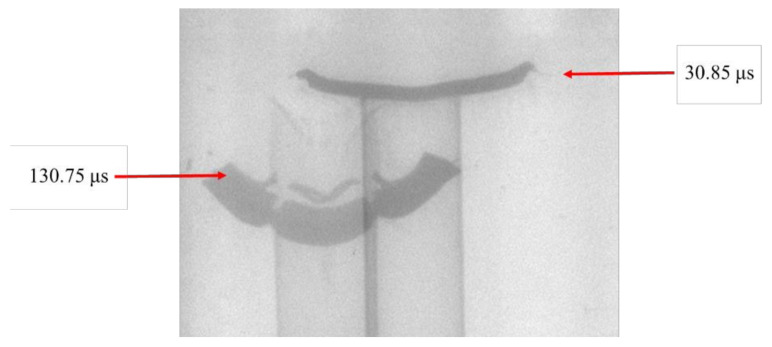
Flash X-ray experiment images at a typical time.
